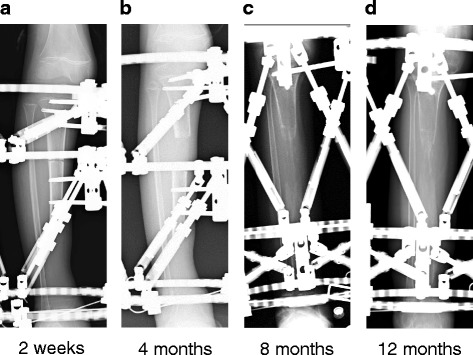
a X-ray 2-weeks post-surgery before starting bone transport showing bone fragment was at same site as immediately after surgery. b At 4-months, and after starting bone transport, the bone fragment was located in the center of the bone defect c At 8-months, morphogenesis of the bone trunk was observed, with the bone fragment in contact with the distal end of the tibia. d At 12-months, reformation of the bone trunk was noticeable, but fusion failure with the distal end of the tibia and the bone fragment was observed
